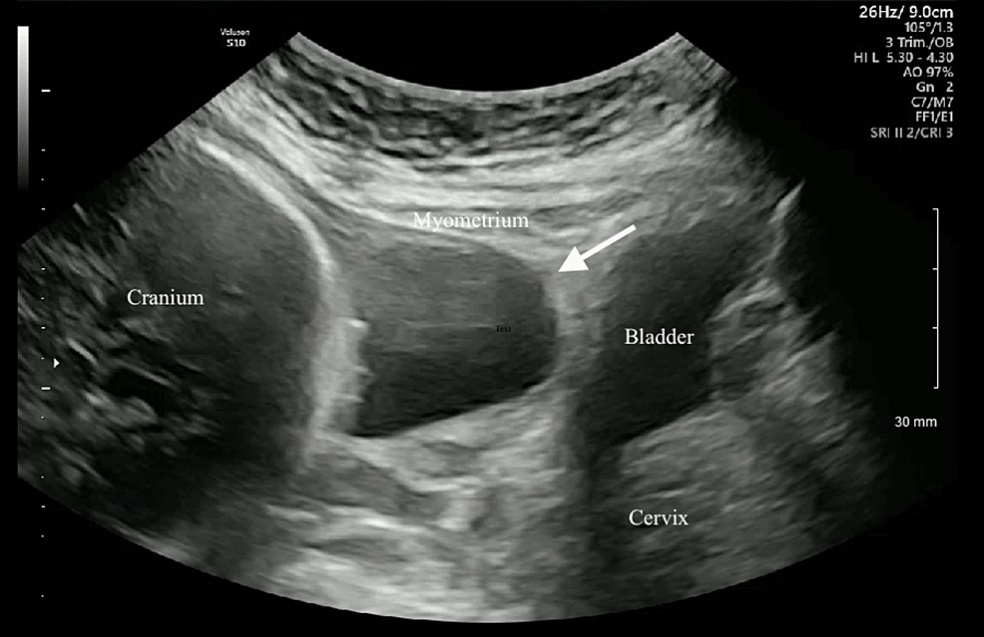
Transabdominal sonographic image of the lower uterine segment showing the presence of a thin myometrium that tapers off and herniation of the amniotic sac toward the bladder in the sagittal view.
